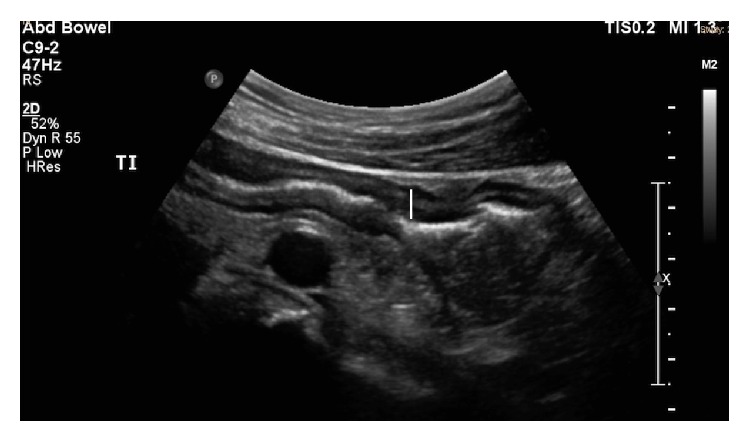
First suggestion of terminal ileal Crohn's disease identified on sonography, with thickened distal ileum running over the hypoechoic iliac artery. The white line marks the thickened ileal wall with echogenic or white air in the lumen.
